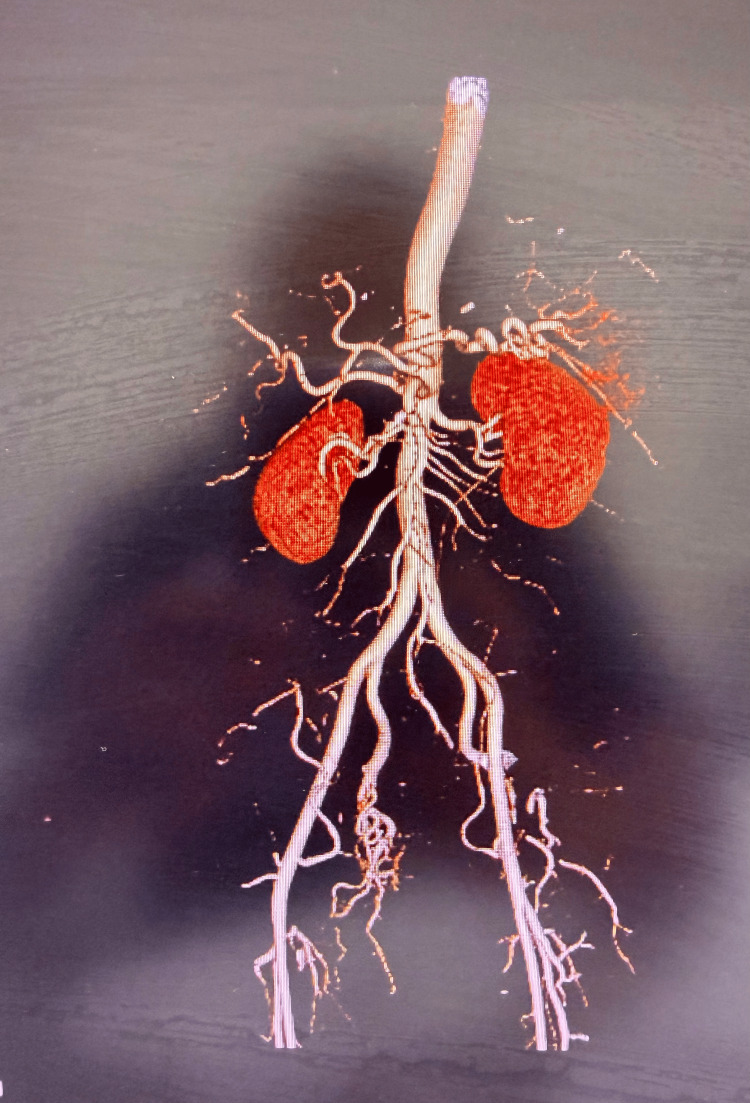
Three-dimensional CT angiographic reconstruction of the abdominal vasculature. Three-dimensional CT angiographic reconstruction demonstrating the abdominal aorta and major visceral arterial branches, including the celiac axis, superior mesenteric artery, and renal arteries with preserved arterial patency. CT: computed tomography.
